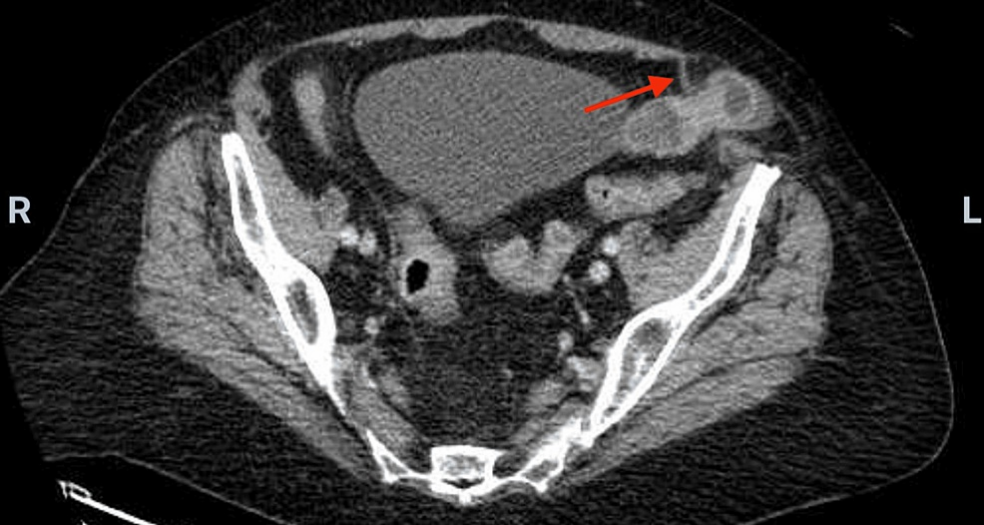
Abdominal CT scan with IV contrast The red arrow indicates the spigelian hernia, sized at less than 2 cm
